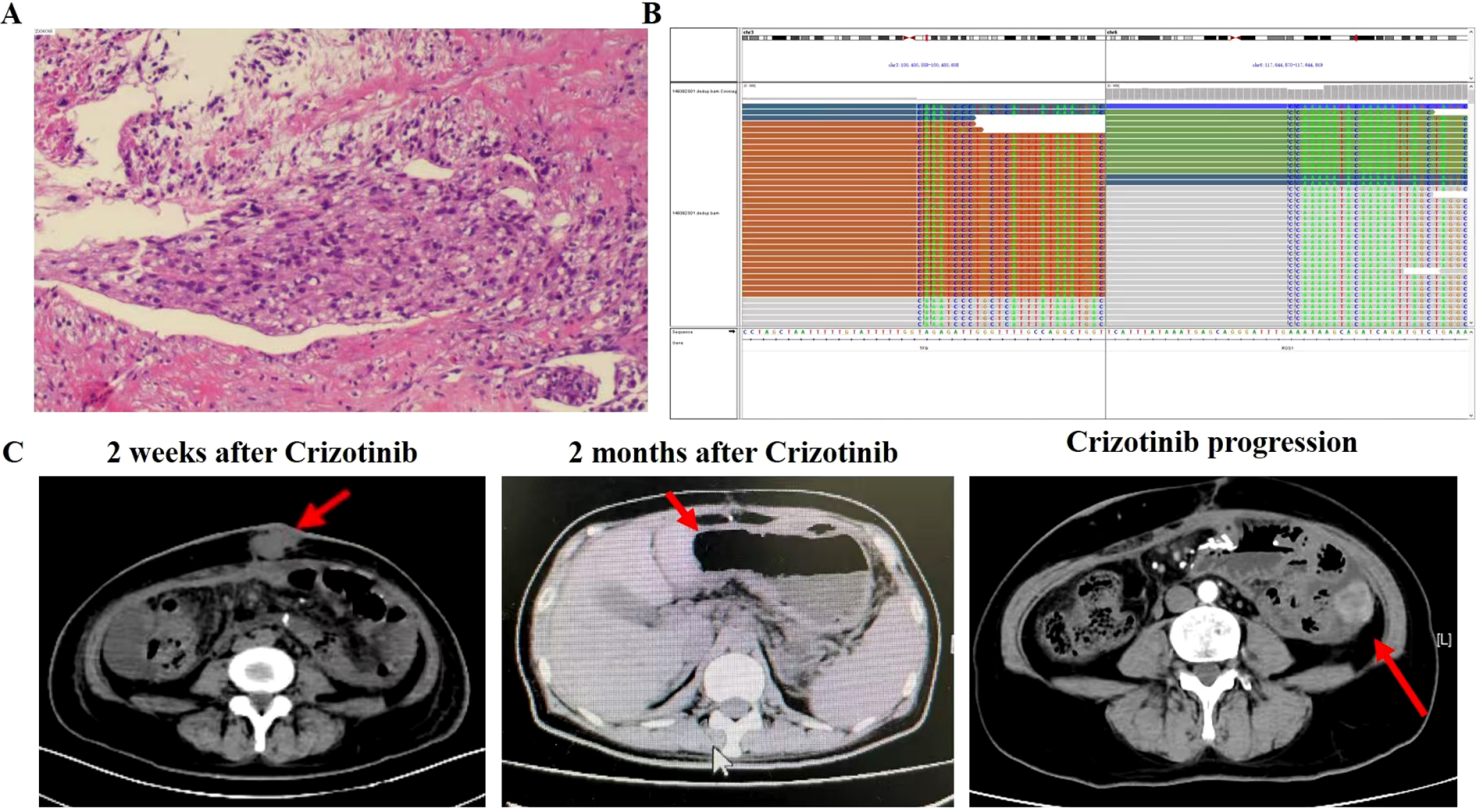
Patient disease diagnosis and treatment of the patient’s disease. (A) Pathologic diagnosis result. (B) Next-generation sequencing results showing break point of *TFG-ROS1* fusion. (C) CT images of lesion changes after crizotinib treatment, as well as new lesions in the left colon as treatment progressed. The red arrow indicates the tumors.
